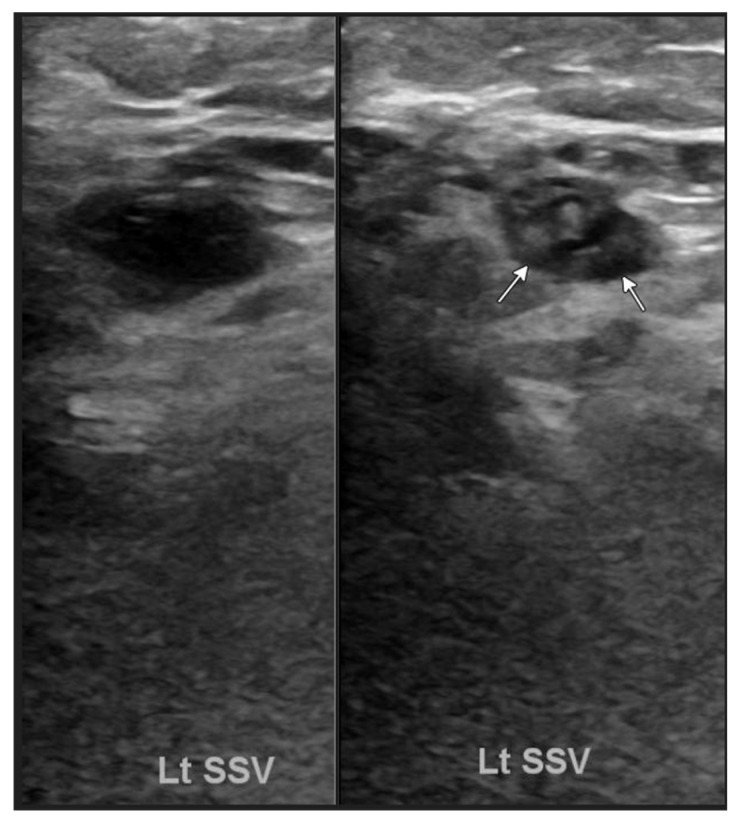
Ultrasound of the left lower extremity with and without compression applied demonstrates a nonocclusive superficial venous thrombus in the left small saphenous vein (arrows).
